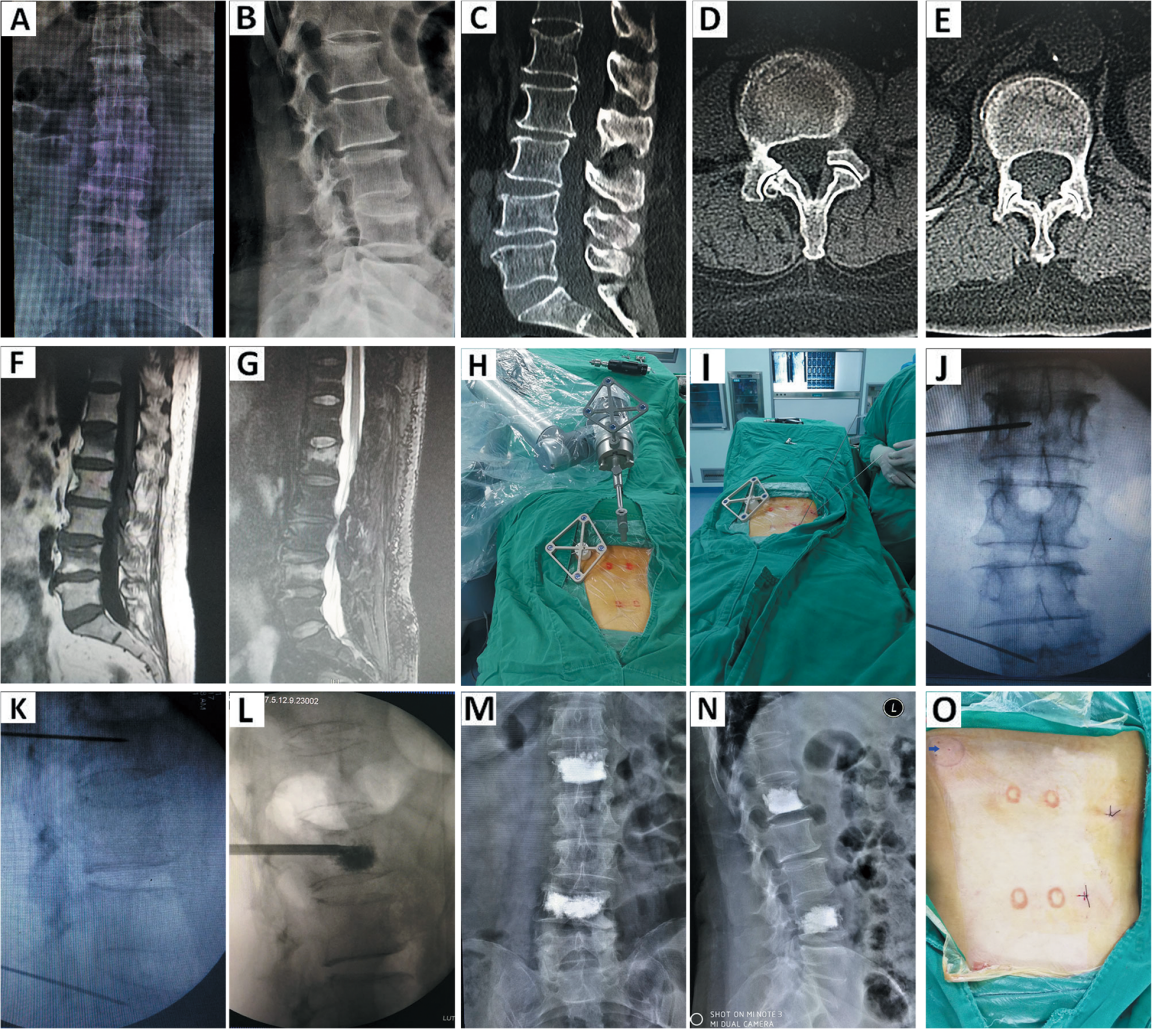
Robot-assisted PVP or PKP and postoperative image verification with improved minimally invasive tracer fixation. The Patient was diagnosed with lumbar osteoporotic fracture(L1 and L4): a-b. preoperative anteroposterior and lateral radiographs. c-e. preoperative sagittal and axial CT. f-g. Preoperative sagittal MRI showed abnormal signal of bone marrow edema(L1 and L4). h. The method of improved minimally invasive tracer fixation. i. Robot-assisted guide wire placement. j-k. The position verification of guide wire. l. Bone cement injection under fluoroscopy. m-n. Postoperative image verification. o. Postoperative wound: Minimally invasive wound was marked with arrow(→)
